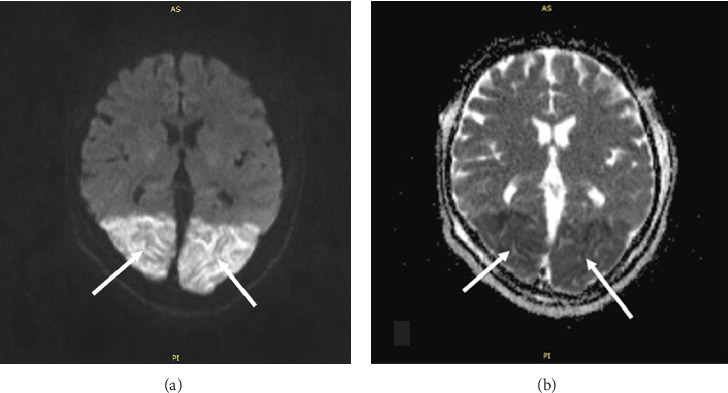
DWI (a) ADC and (b) sequences indicating ischemic infarction by diffusion restriction (white arrows).
